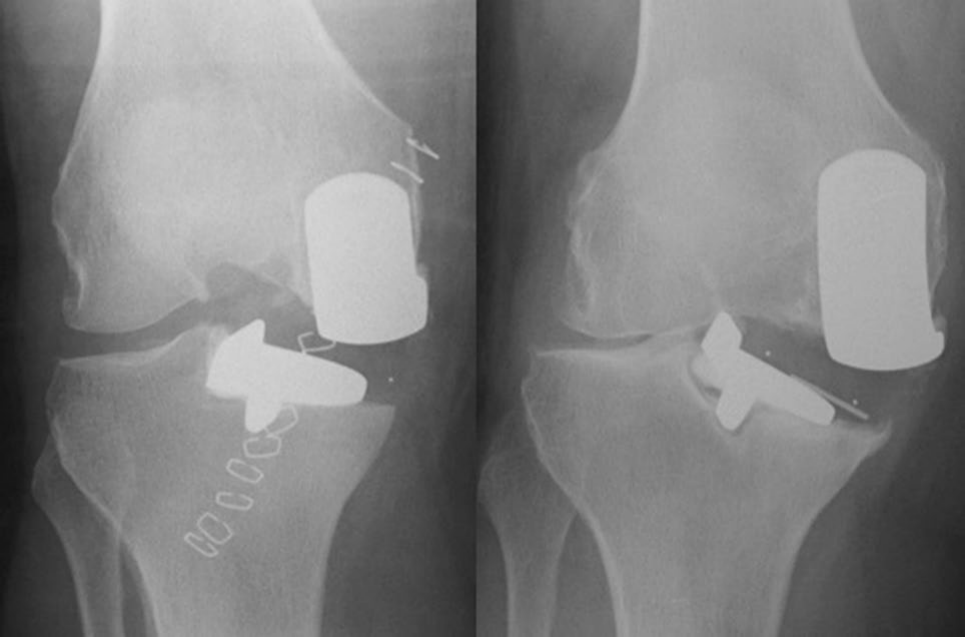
A post-primary and pre-revision radiograph of a poorly positioned tibial component leading to tibial loosening
